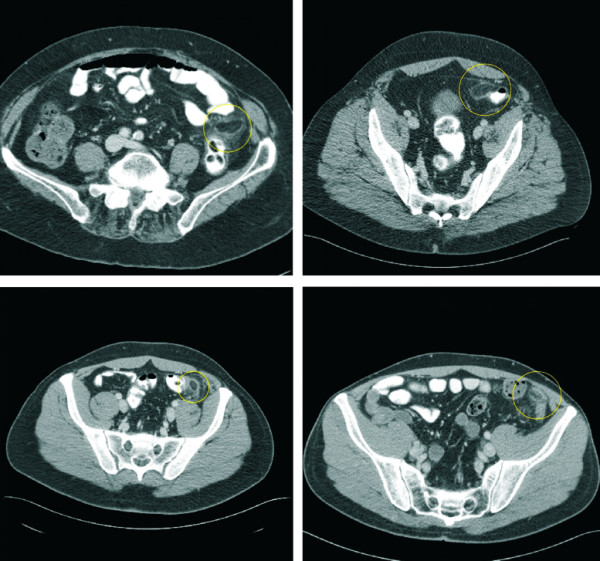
Abdominal CT scan demonstrating the horizontal section of four different patients with EA (circle).
